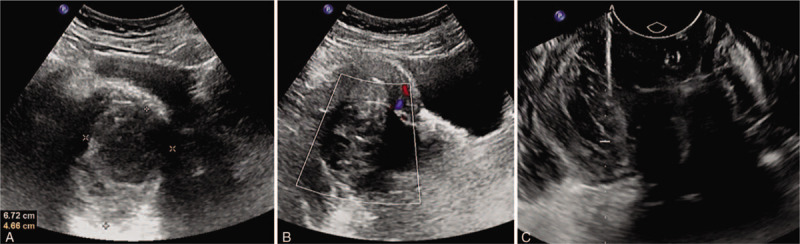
US examination of the pelvis. (A) Color Doppler showed a 6.72 × 4.66 cm hypoechoic or anechoic mass, with no obvious blood flow signal. (B) Color Doppler showed a 6.26 × 5.00 cm well-defined heterogeneous hypoechoic mass in the medial aspect of posterior uterine wall, with a little blood flow signal in peripheral of mass. (C) Transvaginal ultrasound-guided puncture biopsy from vaginal mass. US = ultrasonography.
